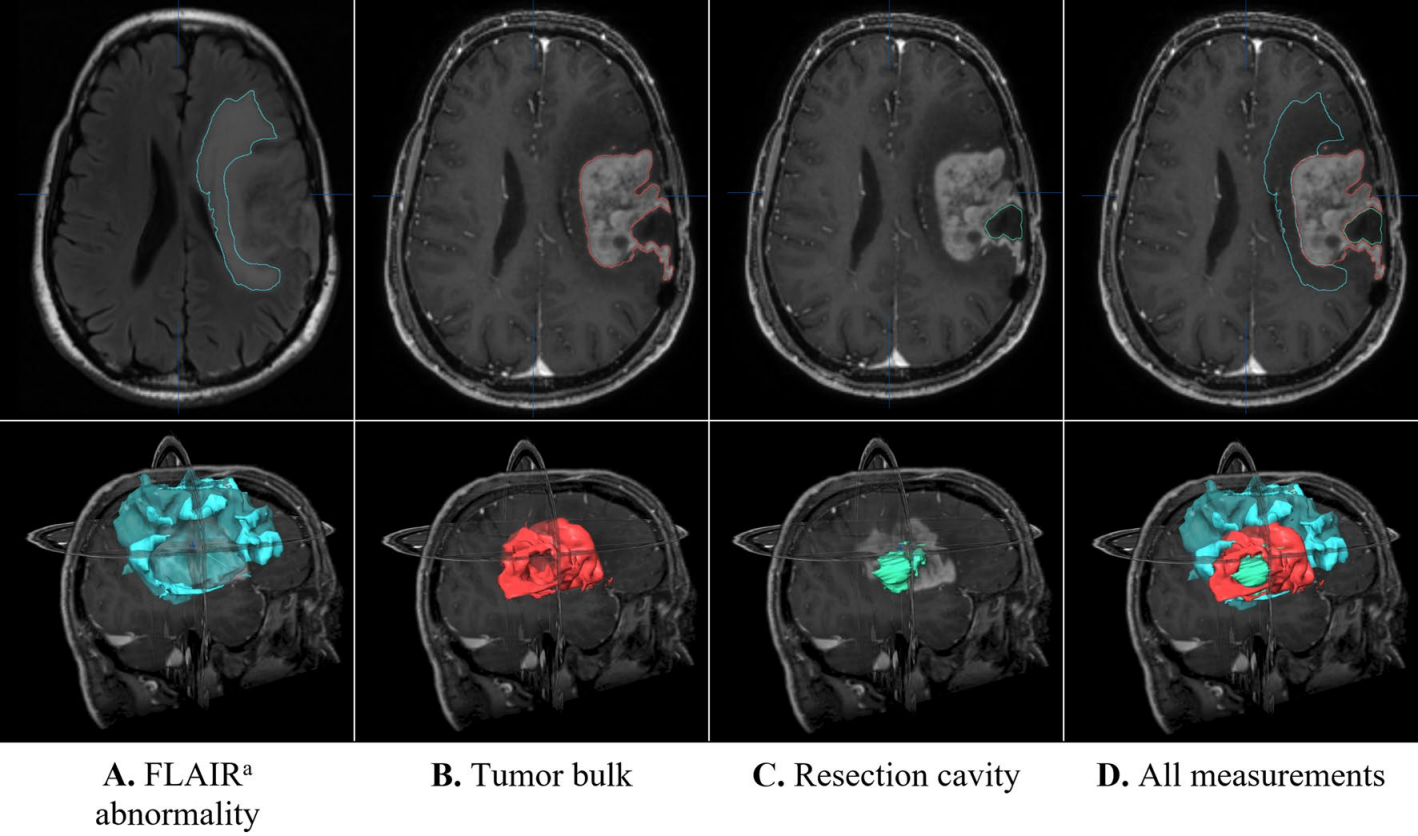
Volumetric measurements of postoperative MRI imaging. All volumetric measurements were done by using BrainLab IPlannet 3.0 software (Brainlab AG, Munich, Germany). Pre-operative measurements of FLAIR^a^ T2-weighted (FLAIR^a^ abnormality) and contrast-enhanced T1-weighted magnetic resonance imaging (tumor bulk and resection cavity) in a patient with recurrent HGG with prior tumor resection who underwent a repeat resection with Gliadel wafers. ^a^T2-Weighted fluid-attenuated inversion recovery.
